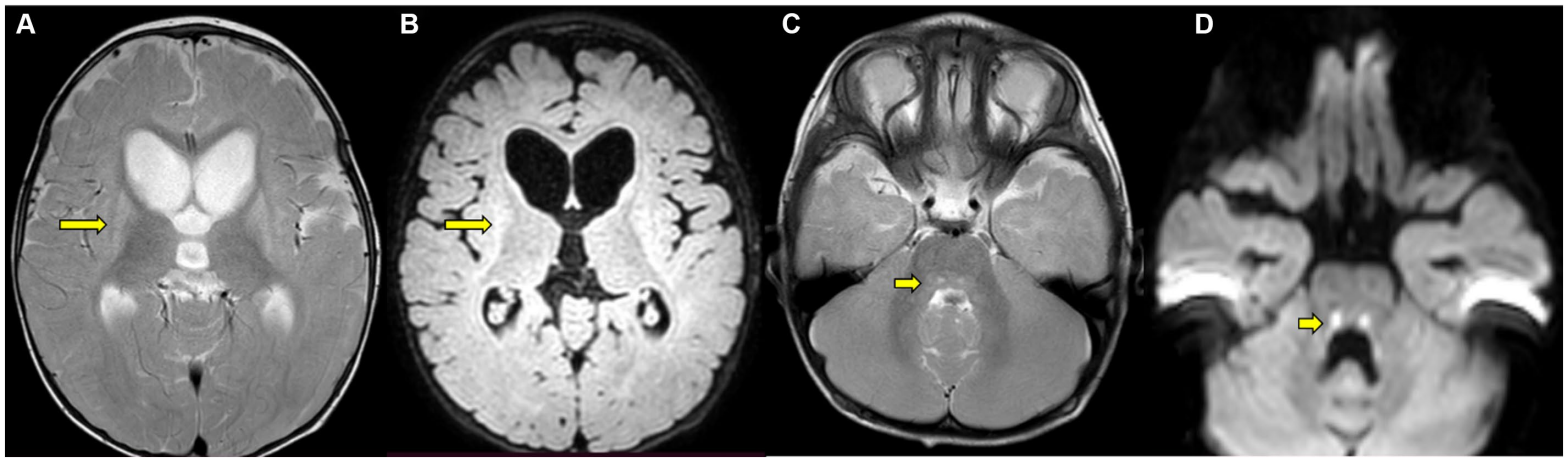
(A–D) MRI brain at 1 year of age in case 4. (A) Axial T2 and (B) FLAIR sections at the level of the basal ganglia show atrophy and hyperintensity in the head of the caudate nucleus and lentiform nuclei bilaterally (arrow) with hydrocephalus (C) T2-axial section at the level of the pons shows hyperintensity in central tegmental tract with (D) diffusion restriction (arrow).
